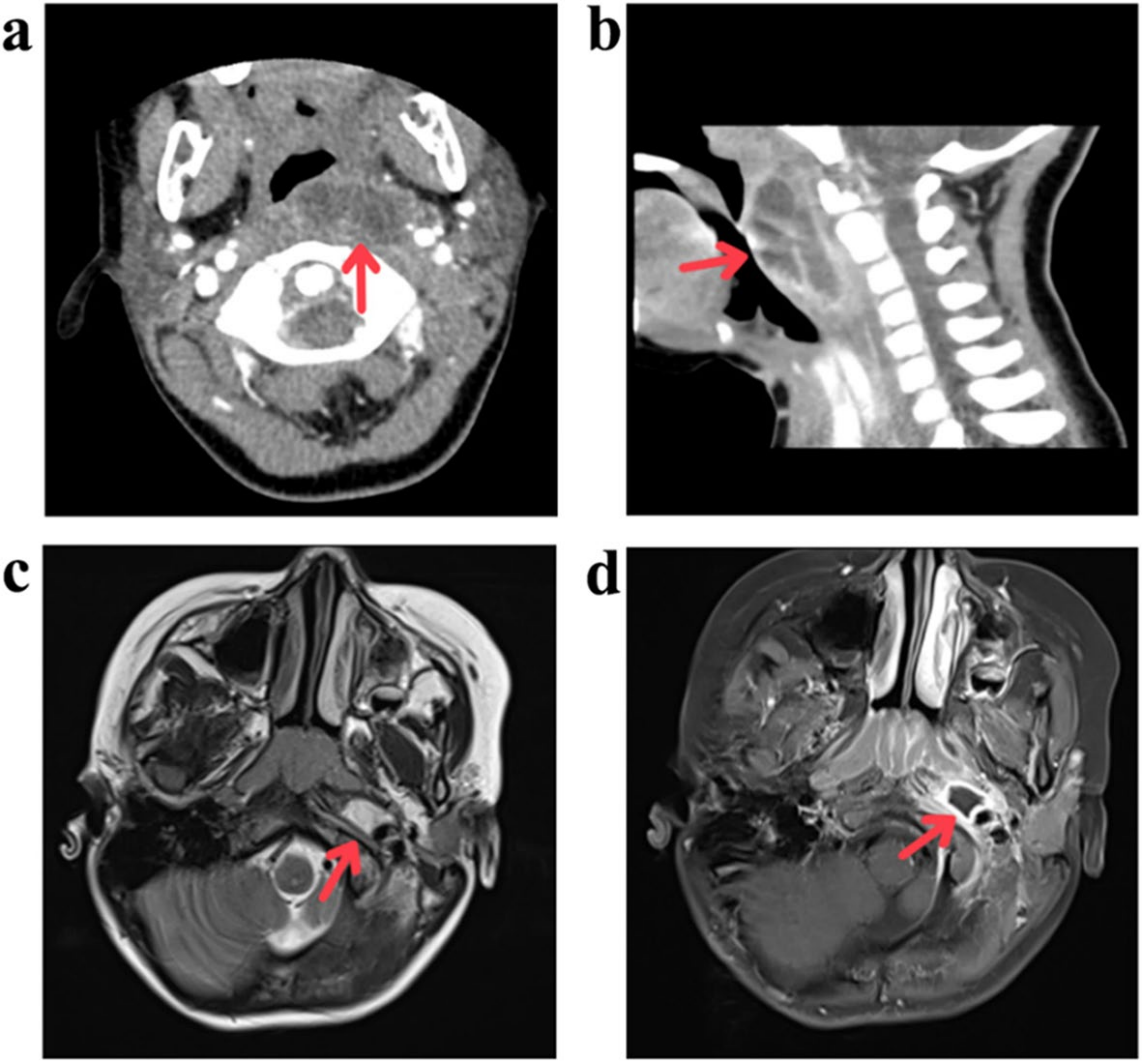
Neck radiological findings of retropharyngeal abscess without KD. a, b Axial and sagittal enhanced neck CT images showing a large collection filling the retropharyngeal space. There is a marked mass effect and ring enhancement. c, d Axial T2fs MR image and axial postGd T1fs MR image showing a left-sided retropharyngeal fluid collection with soft tissue oedema with significant ring enhancement (arrow)
